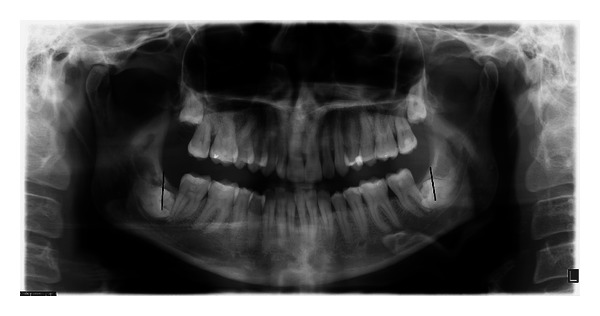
Preoperative panoramic radiograph. The direction of the initial cuts is marked.
